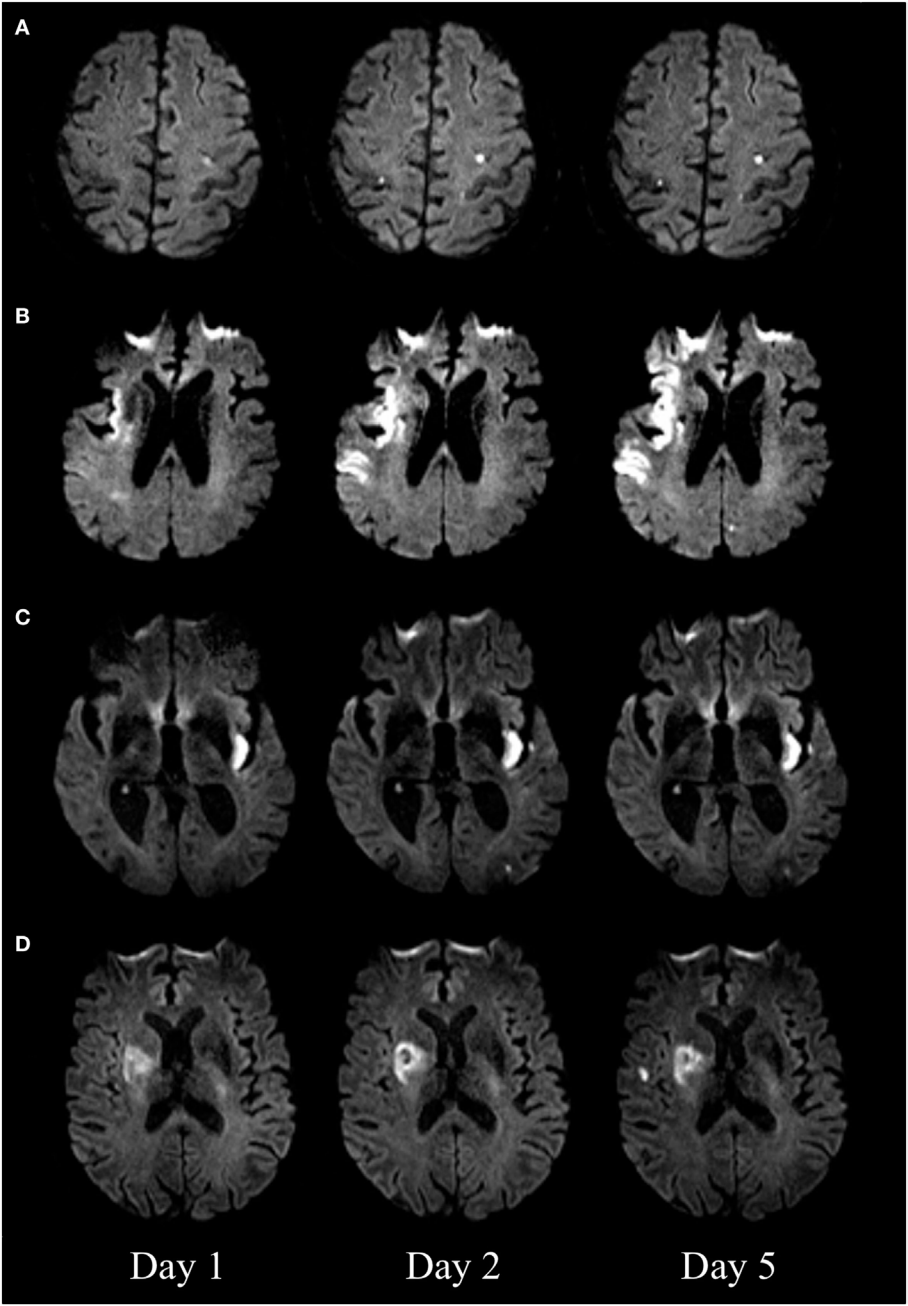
Diffusion-weighted imaging (DWI) of four patients with new DWI lesions. New distant lesions, appearing on day 2 (A) and day 5 (B). New local lesions appearing on day 2 (C) and day 5 (D).
